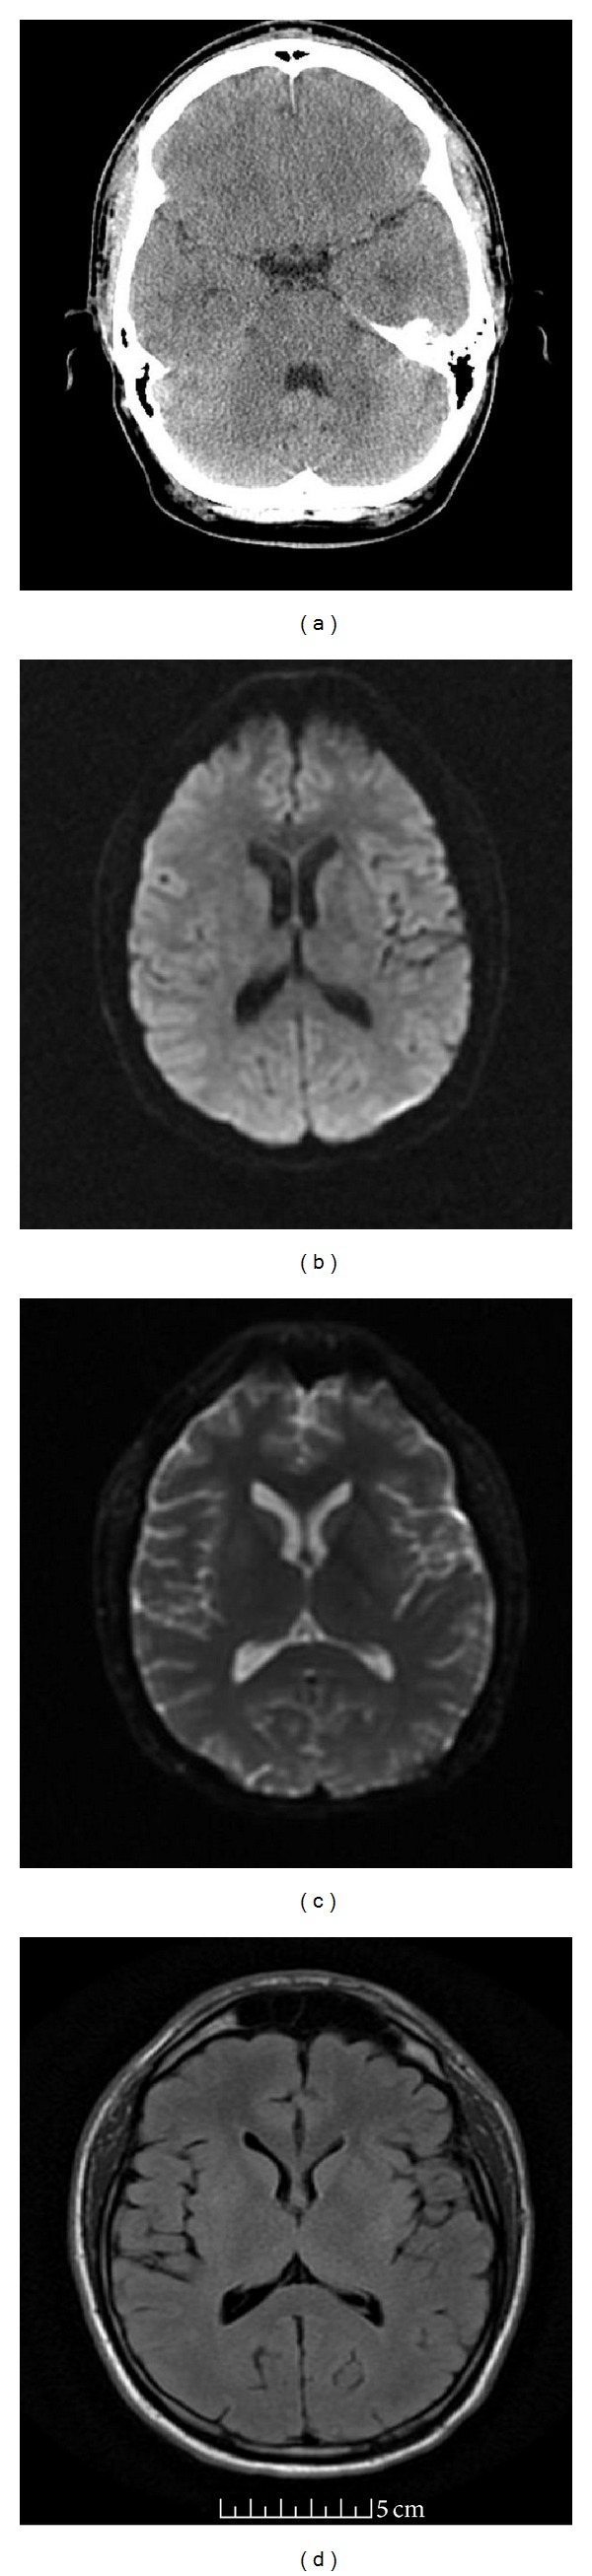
CT scan of brain without contrast was unremarkable (a) and axial MRI of the brain (DWI, ADC map, and FLAIR, resp., (b), (c), and (d)) at the level of basal ganglion was unremarkable.
